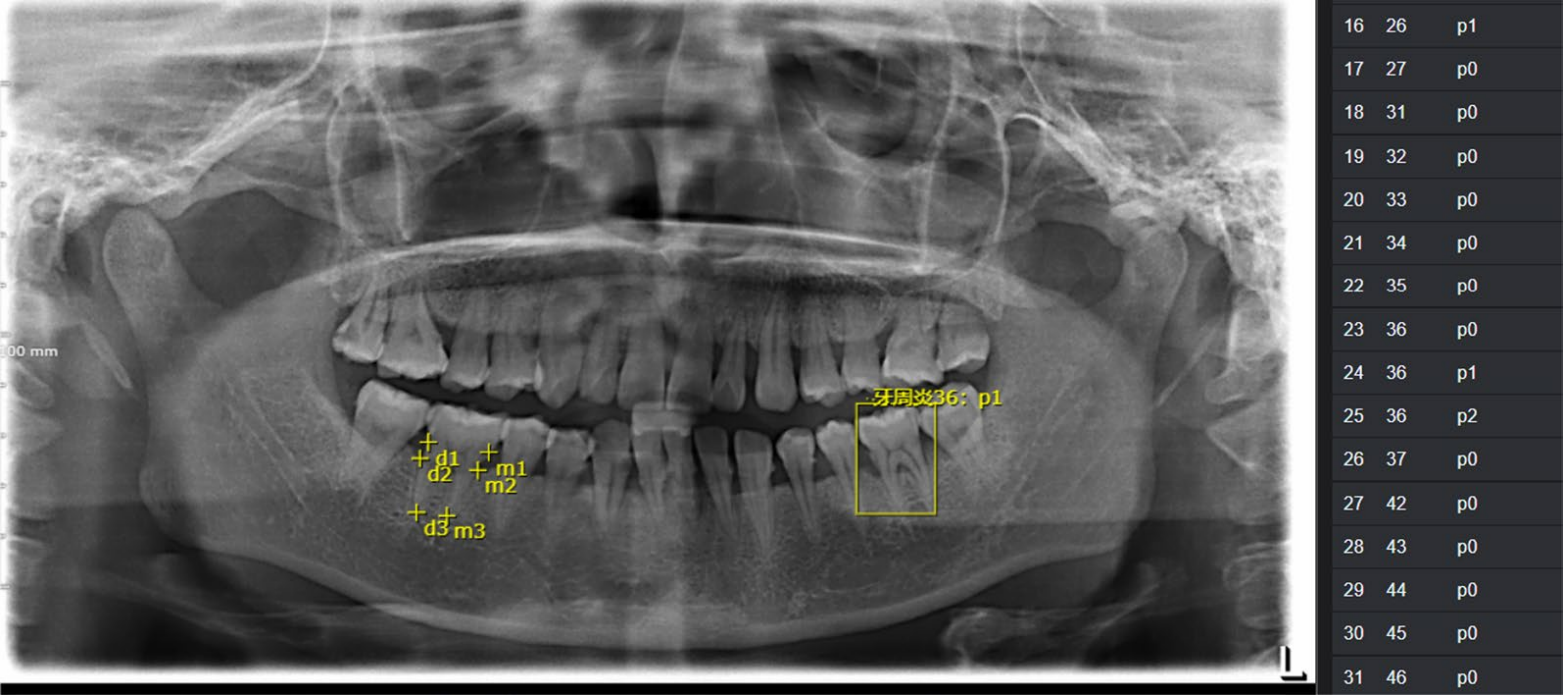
Labels on the key points of each tooth and a tooth with vertical alveolar reduction or furcation lesions on the panoramic radiographs (mandibular first molars were taken as examples) are shown
